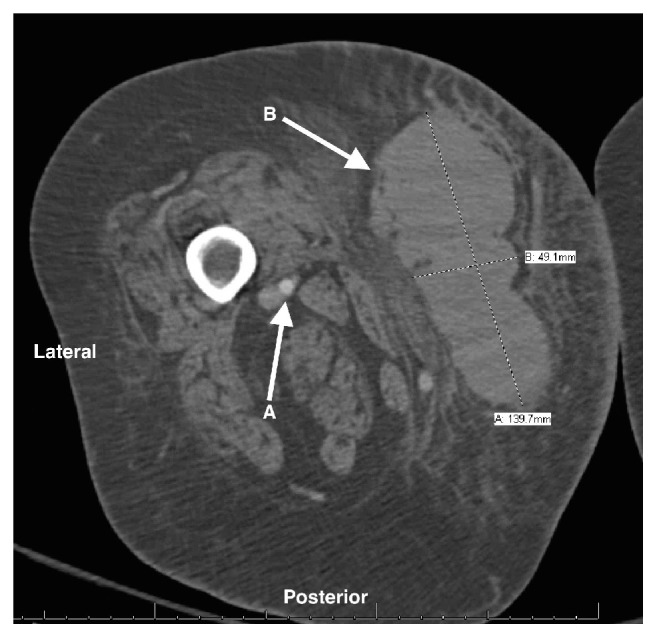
CT of the patient's right thigh showing the superficial femoral artery (A) and 5 × 14 cm hematoma (B).
